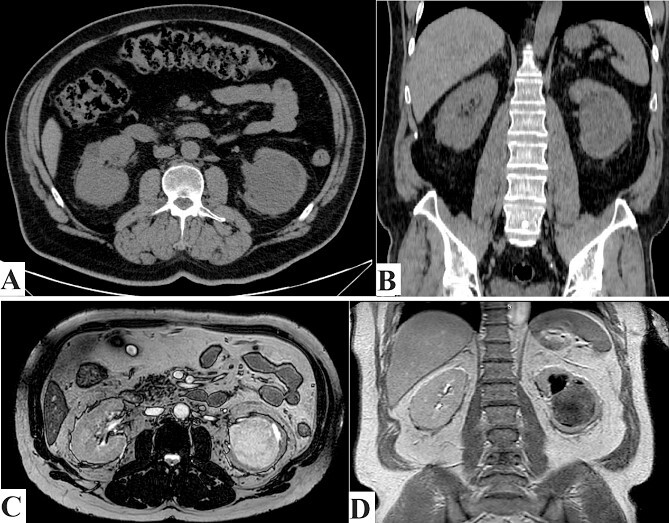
(A) CT scan axial section and (B) CT scan coronal section. Showing left kidney well-defined rounded soft tissue density mass lesions measuring about 6.7 cm in the lower aspect of the renal medulla. (C) MRI axial section, and (D) MRI coronal section. Showing left kidney large well defined rounded hypointense on T1W and heterogenous hyperintense on T2W images focus seen in the lower aspect of the medulla of the left kidney measuring about 6.8 cm approximately, resulting in mild calyceal dilatation.
